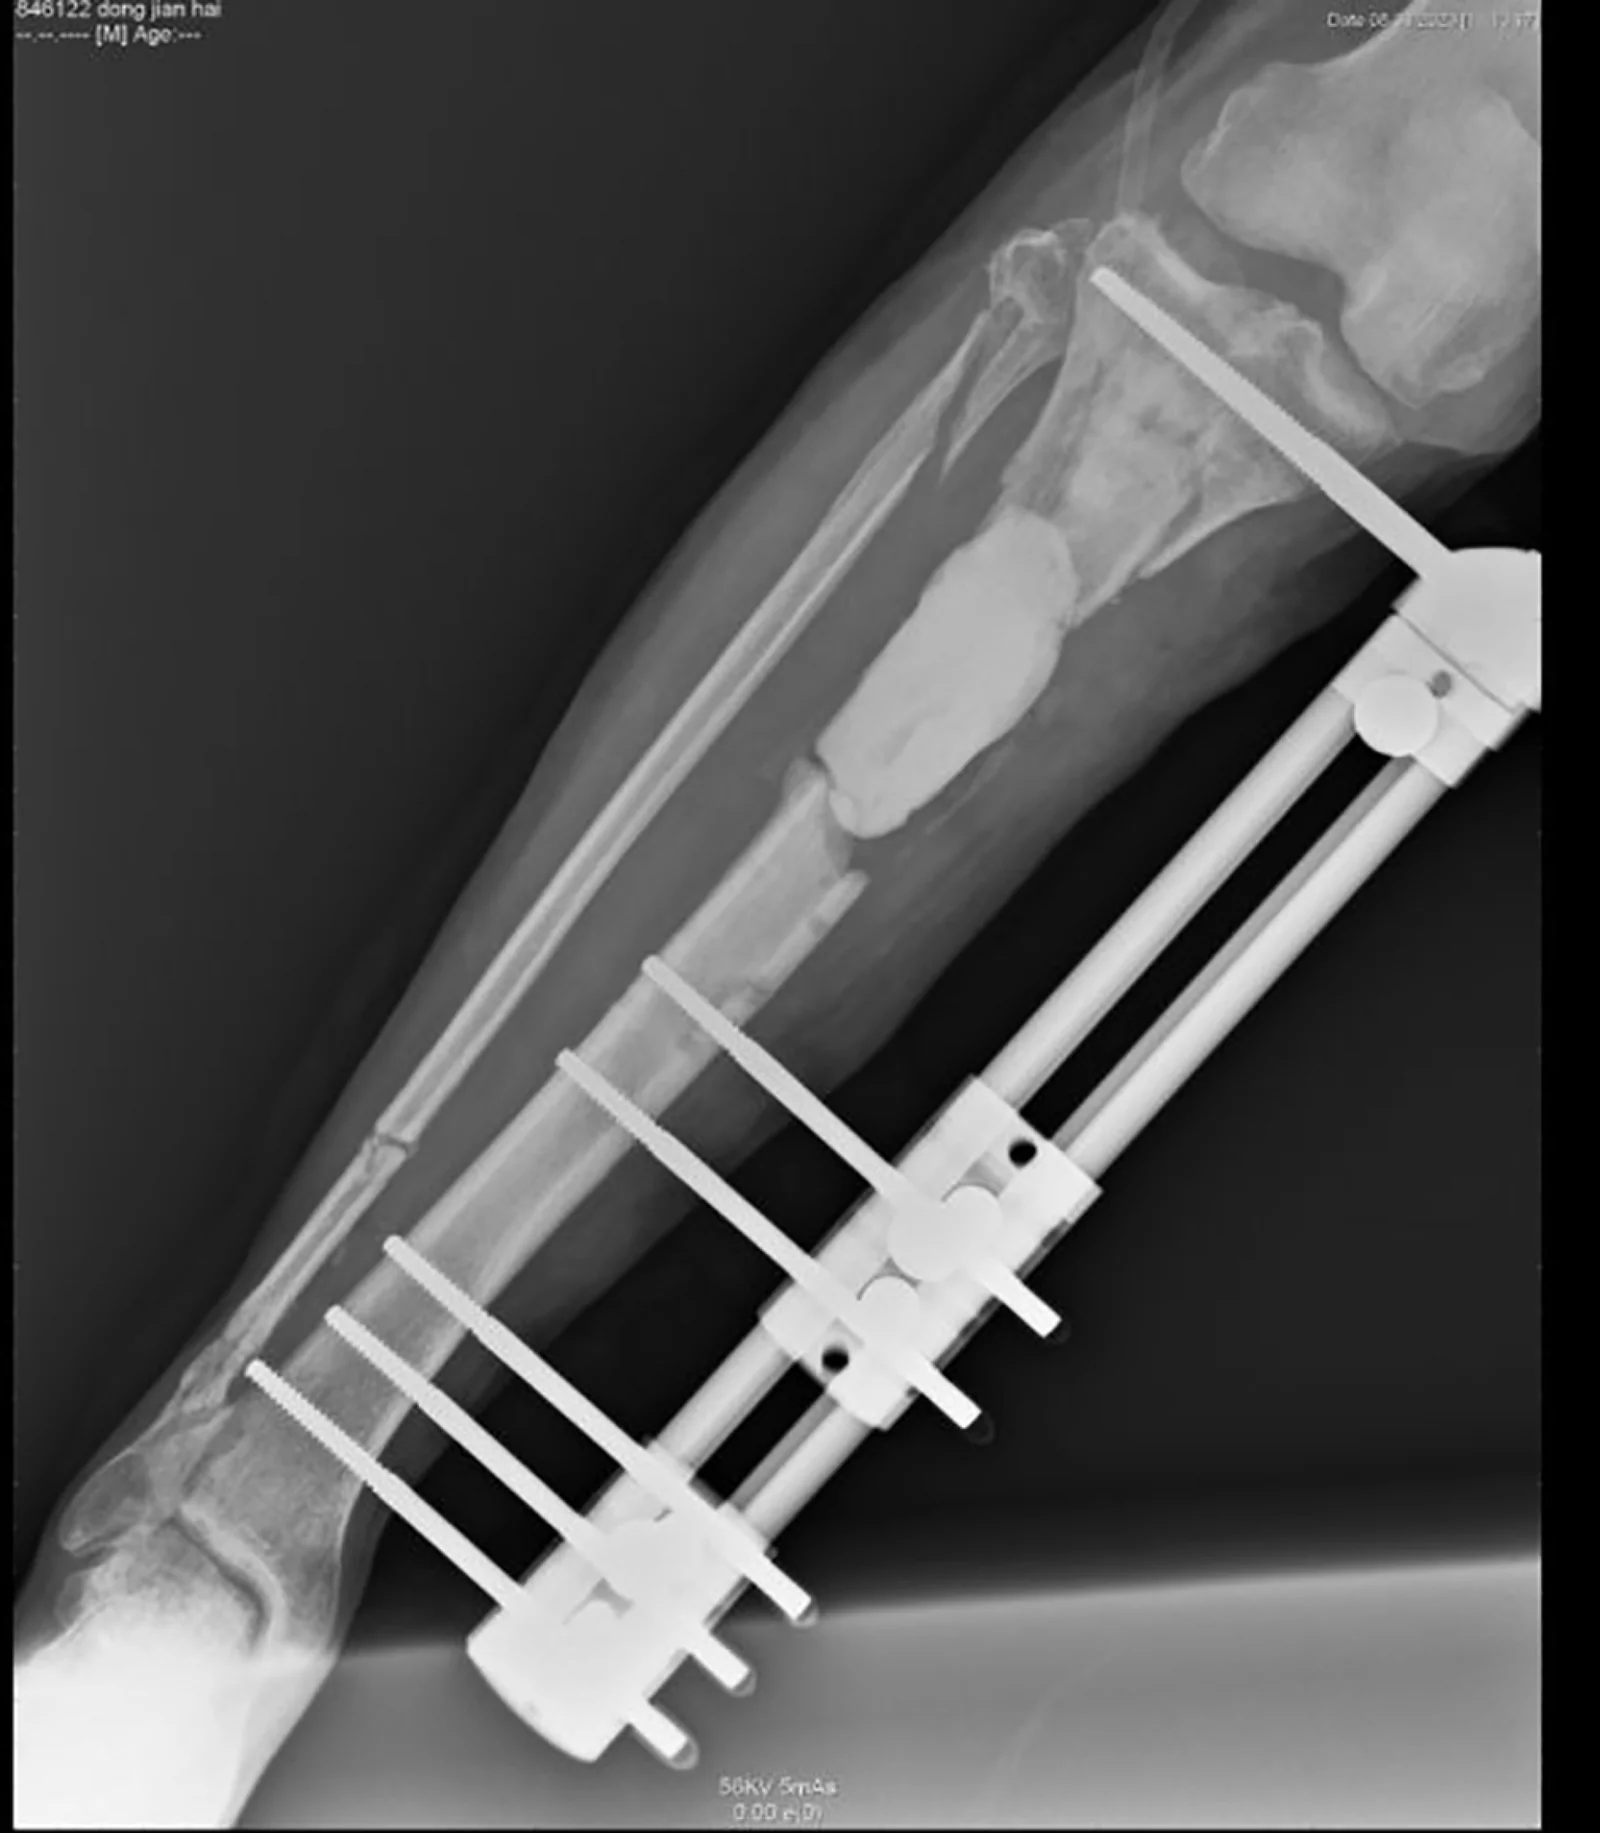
Radiographic appearance after the first-stage surgery. An anteroposterior radiograph demonstrates the application of an external fixator for stabilization. The resected 6 cm segmental bone defect has been filled with an antibiotic-loaded bone cement (ALBC) spacer, which serves to locally elute antibiotics and maintain soft tissue tension.
